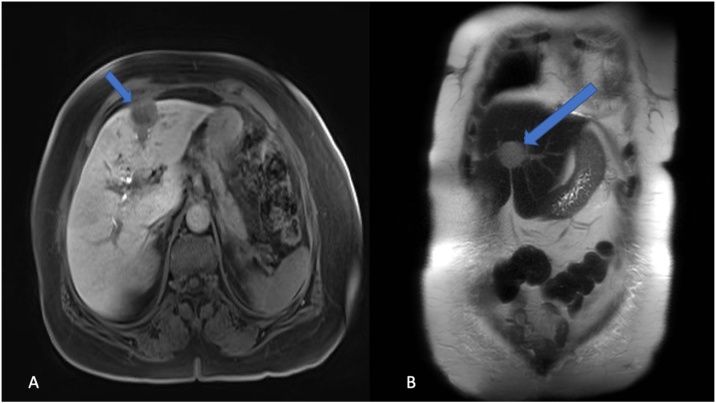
A and B: MRI of abdomen showed two left hepatic lesions located between the medial and lateral portions of the left hepatic lobe (segment IV and II/III), the largest measuring 2.7 × 2.4 × 2.8 cm, and highly suspicious of colorectal liver metastasis.
